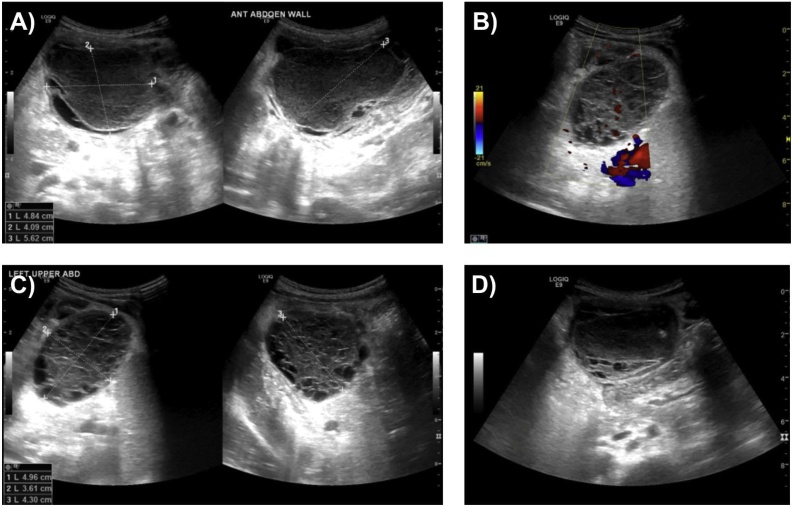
Abdominal ultrasonography (A–D) revealed a well-defined, multiloculated, cystic lesion seen in the epigastric region and extending into the left upper quadrant. The largest locule measured about 4.8 × 4.0 × 5.6 cm seen in the epigastric region containing swirling debris suggesting of an organized abscess secondary to infected mesenteric cyst or a lymphangioma.
